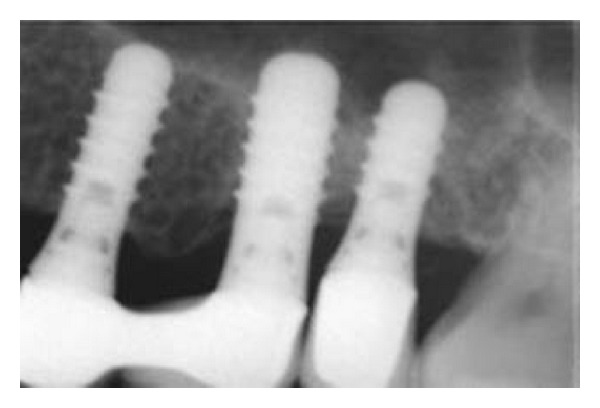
Periapical radiograph after 12 months of prosthetic loading showing a stable clinical situation in the area around the apex of the implants.
